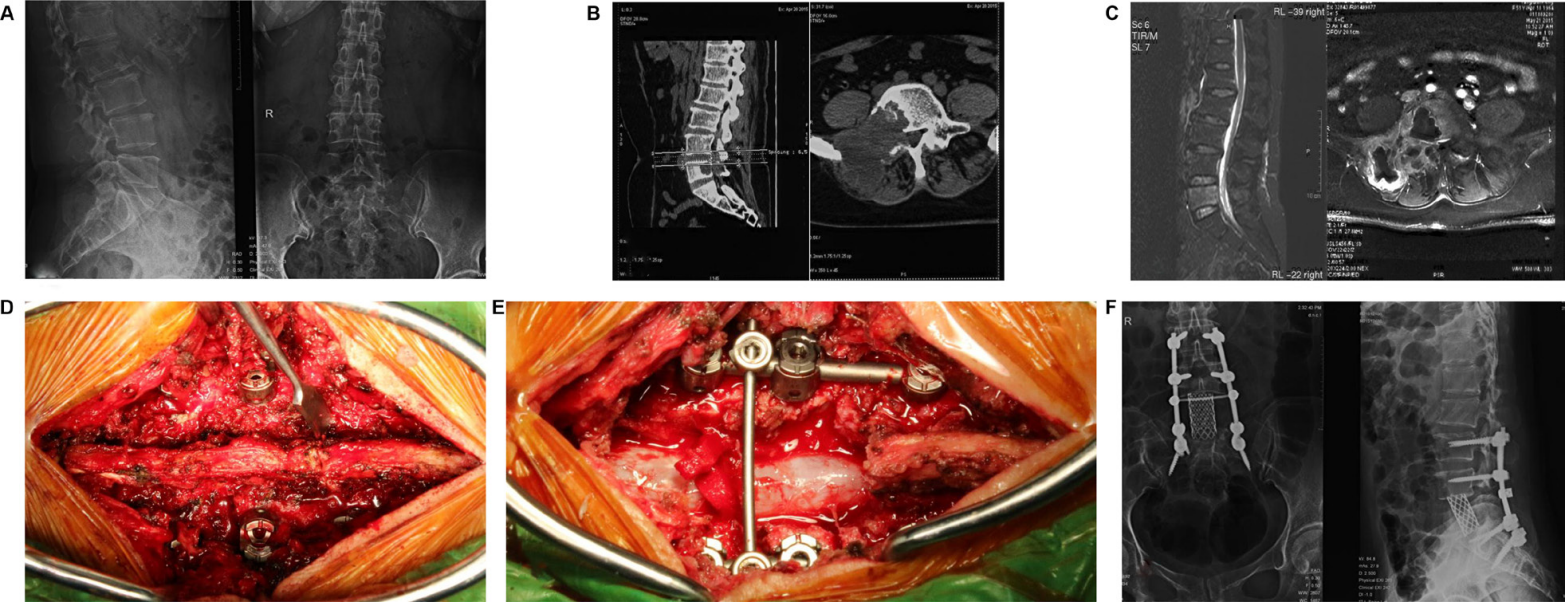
A female patient suffering low back pain for 3 months was made gross total resection (GTR) surgery in Changzheng Hospital Orthopedic Oncological Center (CHOOC) and was pathologically diagnosed as bone Giant Cell Tumor (GCT) (A) the pre-surgery X-ray imaging was shown; however the typical “soap bubble changes” was not obvious. (B) bone erosion of right part vertebral body was obviously revealed by the computer tomography (CT scan). (C) the Magnetic Resonance Imaging (MRI) indicated that the lesion showed low-intensity signal on T1-weighted image and high-intensity signal on T2-weighted image. (D and E) a gross total resection surgery was conducted; the vertebral body and appendix were removed meanwhile the spine was reconstructed by screw-rod system. (F) the post-surgery X-ray imaging showed the L5 vertebra was removed and the internal-fixation was solid and successful.
